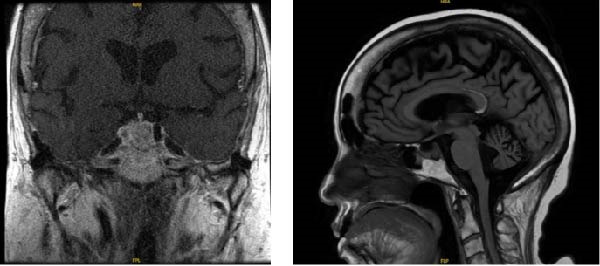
Pituitary MRI shows a 1.3 cm hypoenhancing nodule in the posterior aspect of the sella suggestive of a macroadenoma.
